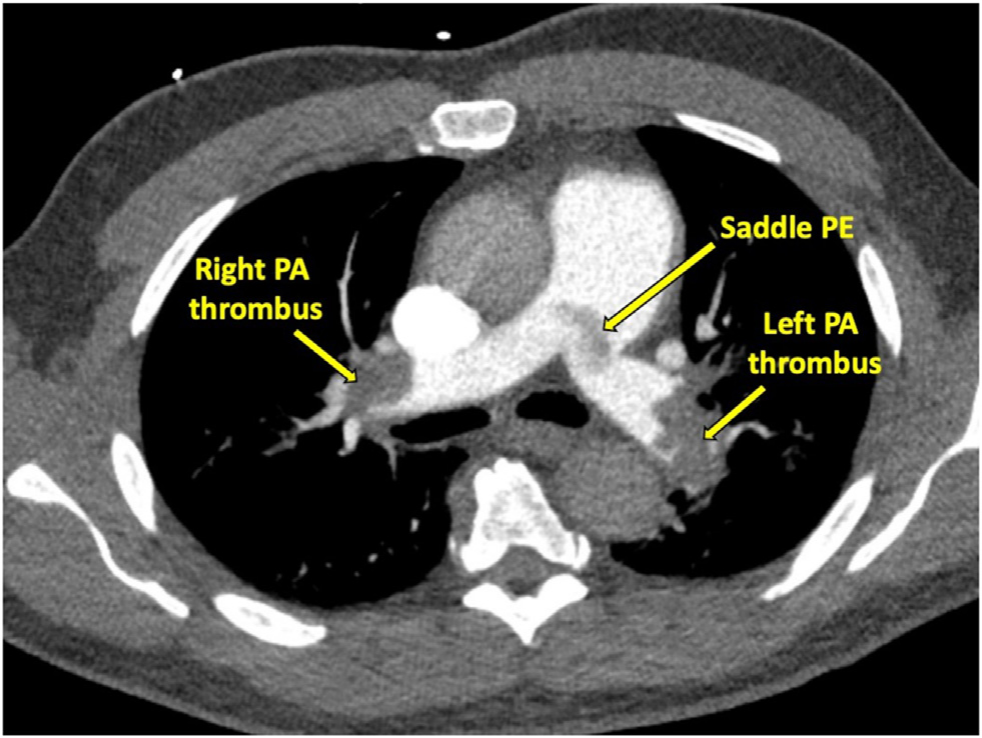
Computed tomography: saddle pulmonary embolism. Bilateral PEs and a saddle PE are demonstrated corresponding to the echocardiographic view. The main PA is dilated to 3.7 cm, suggestive of chronicity.
